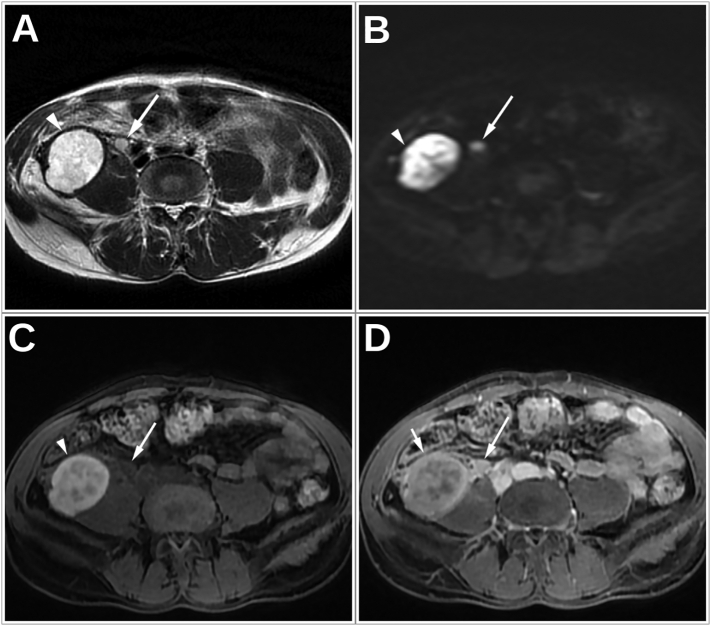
Abdominal MRI images at T2W (A), DWI (B), T1W FatSat before (C) and after (D) gadolinium administration: The ureteral lesion (Arrow) is showing a high signal on both T2W and DWI sequence with a low signal at T1W and an enhancement after Gadolinium administration.
